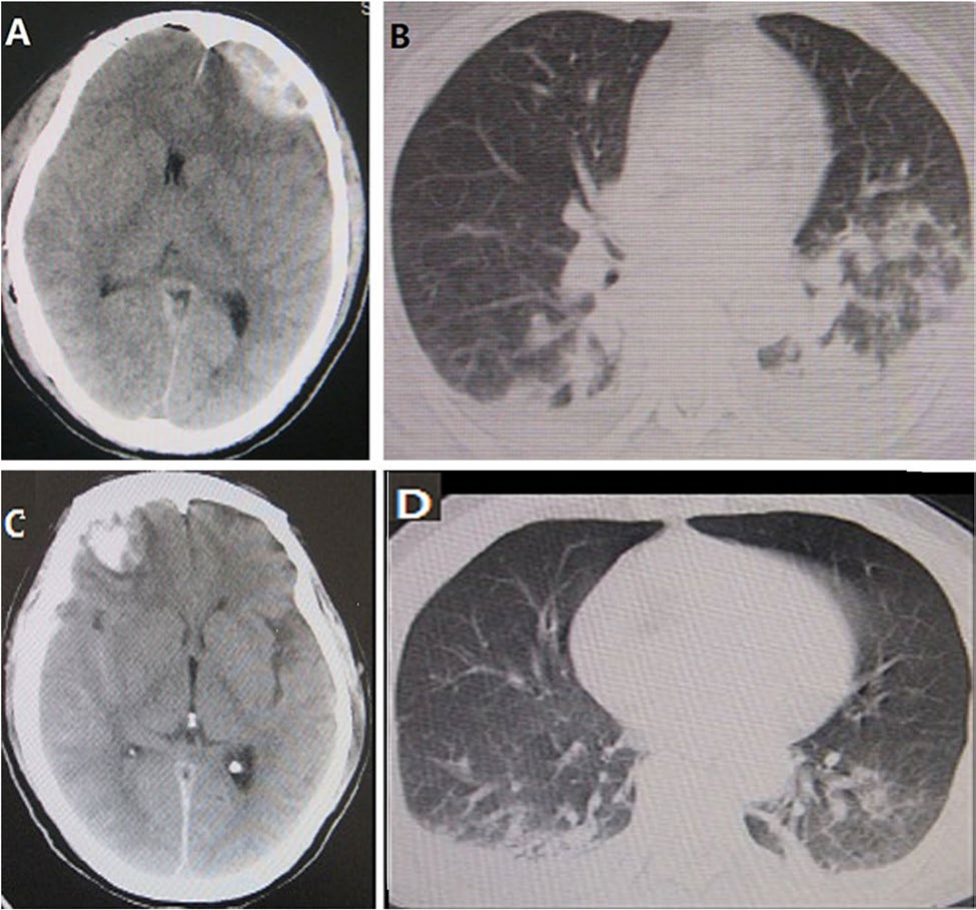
CT images of pneumonia caused brain edema following TBI. Fig A and B represent an adult patient with diffuse or vasogenic brain edema due to pneumonia with later positive sputum culture following TBI. Fig C and D represent an adult patient with diffuse brain edema caused by pneumonia with later positive sputum culture following TBI
